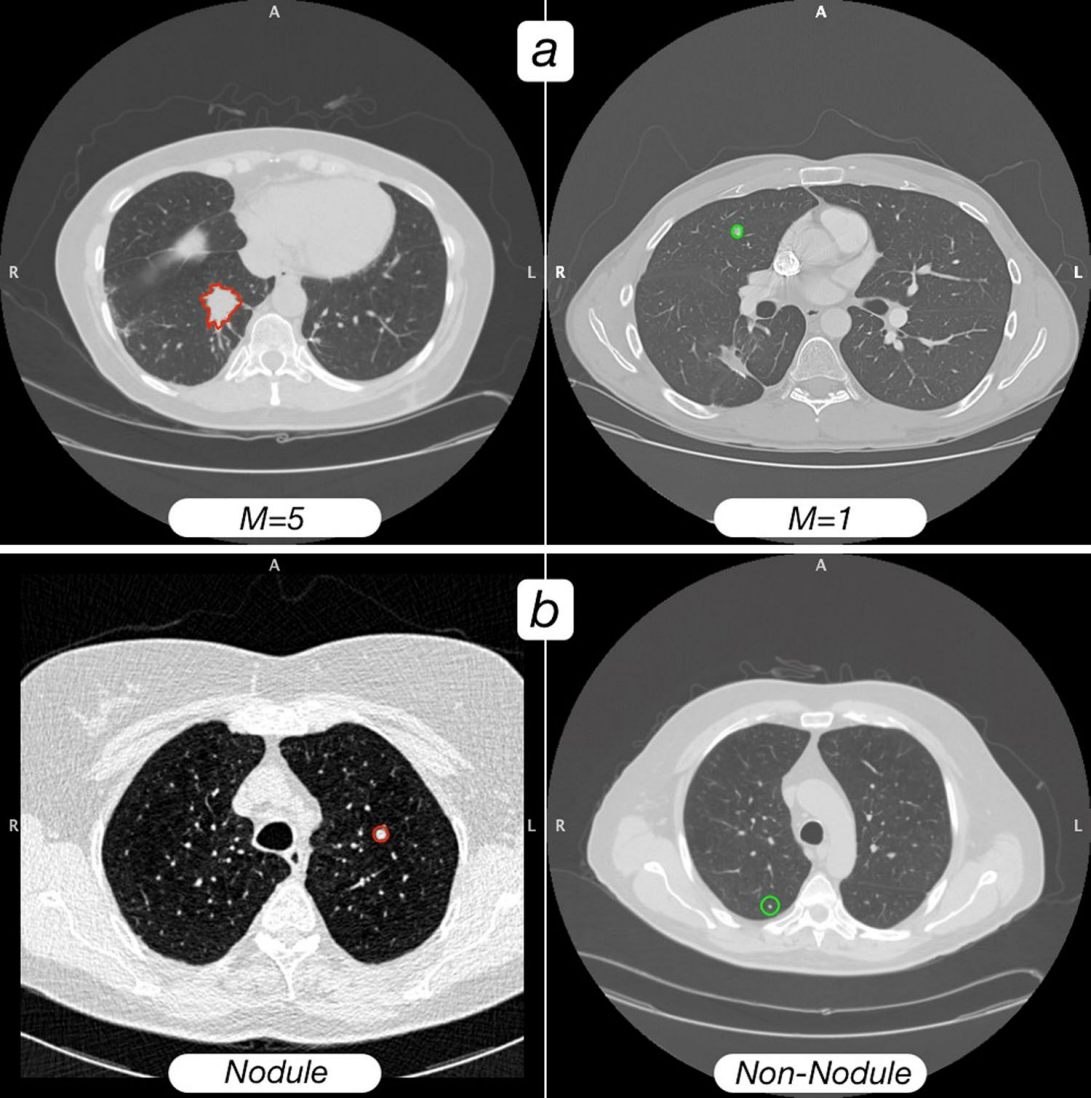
(a) A visual comparison of a likely malignant nodule versus a likely benign nodule. The CT scan on the left (with red ROI outline) was rated at malignancy = 5 (as highly likely malignant nodule) by consensus of the LIDC/IDRI radiologists who rated the nodule; the scan on the right (with green ROI outline) was rated as malignancy = 1 (as likely benign nodule) by consensus of the LIDC/IDRI radiologists who rated the nodule. Both outlines are from the consensus of segmentations provided by the LIDC/IDRI radiologists. (b) A visual comparison of a nodule versus a non-nodule. The left image is a “nodule” (rated malignancy = 3 from LIDC/IDRI) with consensus radiologist segmentation (red), and the CT scan on the right is a “non-nodule,” with computer segmentation (green). Computer segmentations were used for “non-nodules”, as LIDC/IDRI radiologists did not provide segmentations for these regions.
